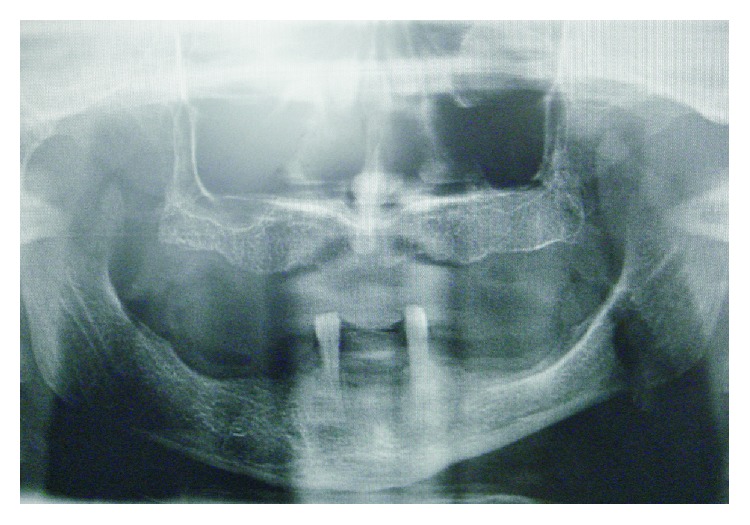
Panoramic radiograph of patient with two poor-prognosed mandibular teeth.
